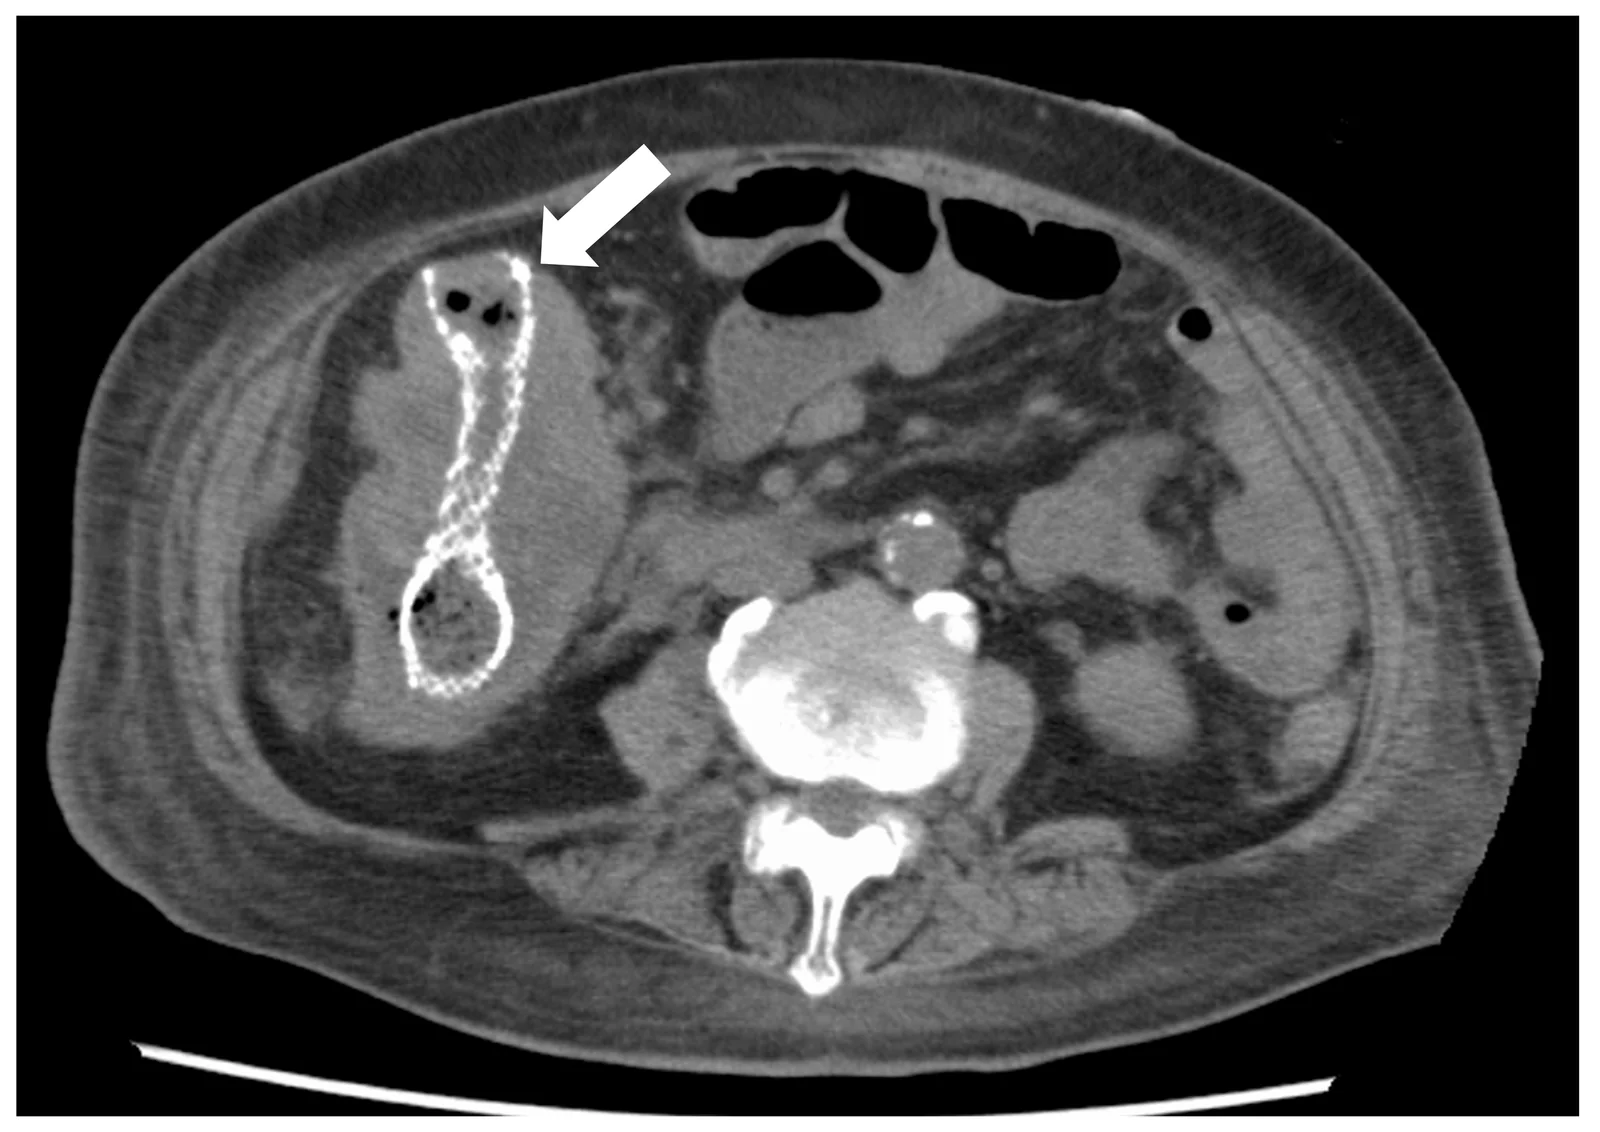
Unenhanced axial computed tomography image obtained on postprocedure day one. Unenhanced axial computed tomography (CT) image obtained on postprocedure day one, showing the uncovered Niti-S colonic self-expandable metallic stent (arrow) correctly positioned at the hepatic flexure. The stent is expanded across the malignant stricture with no evidence of migration or perforation. In direct comparison with the preprocedure CT (Figure 1), the marked proximal colonic dilatation has substantially resolved, confirming successful luminal decompression.
